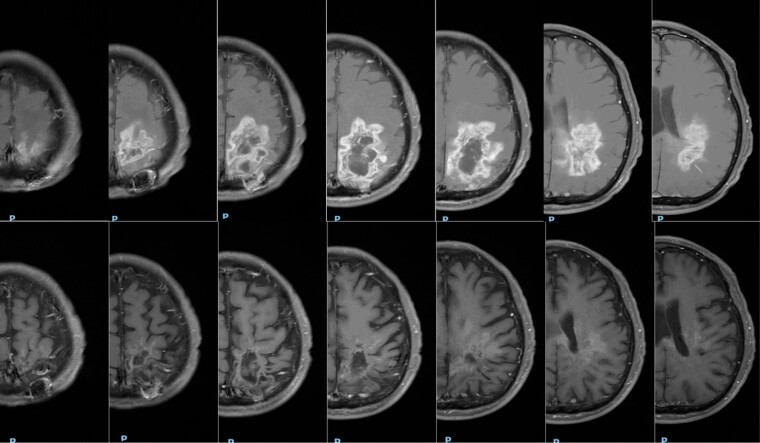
MRI axial images T1 + gadolinium contrast. Top: Baseline: imaging; Bottom: after 6 cycles pemetrexed-bevacizumab-candesartan: 5 months later. The follow-up study shows marked decrease in contrast enhancement and regression of disease.
